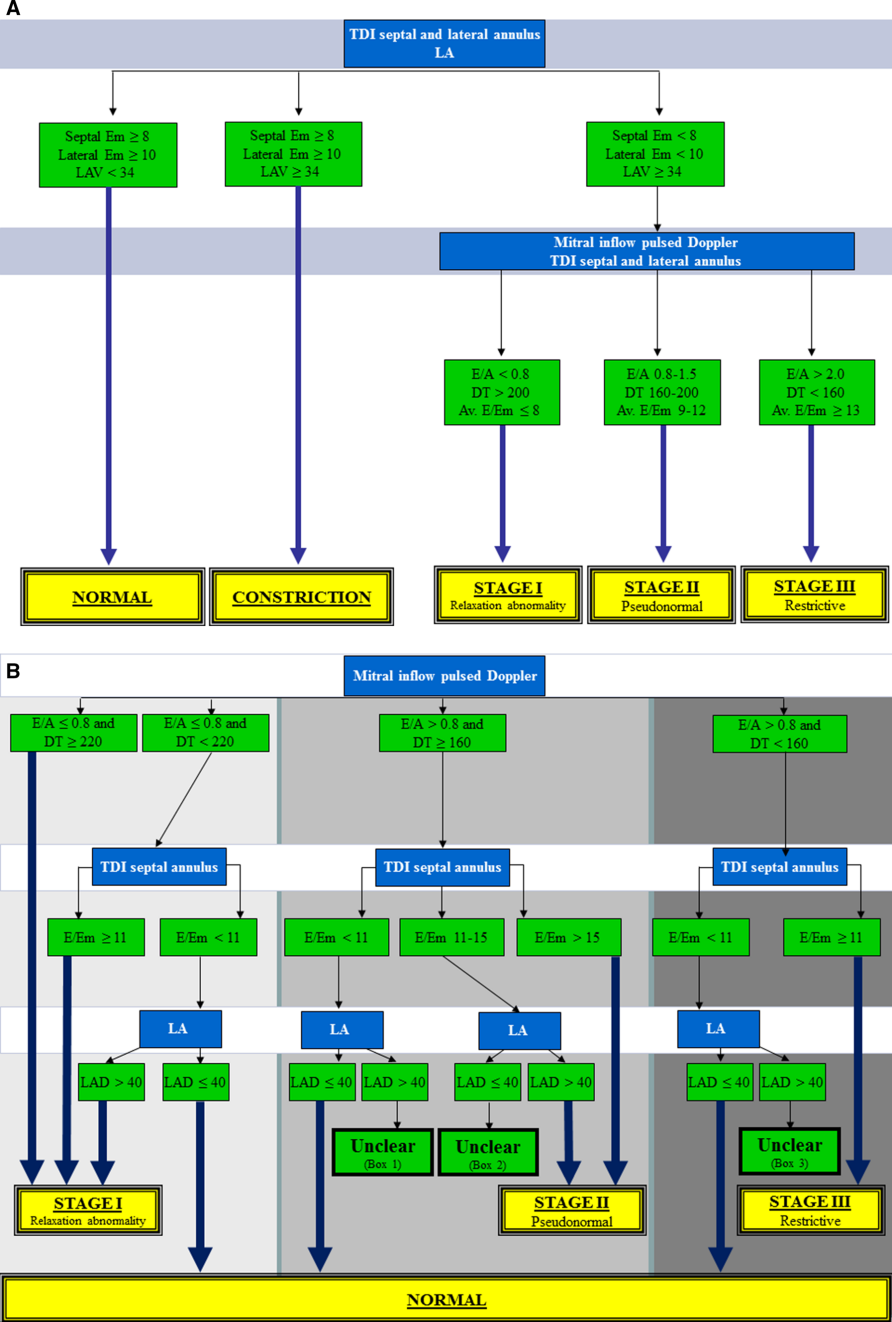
a Grading of left ventricular diastolic function according to the ASE/EAE algorithm, b Grading of left ventricular diastolic function according to TXC algorithm. *E* peak early filling velocity, *A* peak late filling velocity, *DT* E-velocity deceleration time, *Em* velocity of the mitral annulus early diastolic wave, *TDI* tissue Doppler imaging, *LA* left atrium, *LAD* left atrial dimension, *LAV* left atrial volume, *Av* averaged (from septal and lateral measurements)
